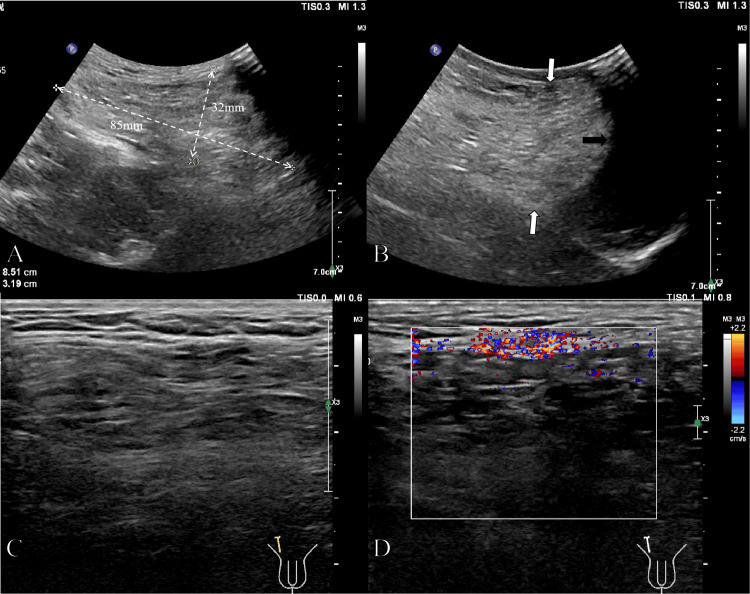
Color Doppler ultrasonography findings. A low-frequency probe was used to evaluate the overall morphology of the hernia sac (A); the inferior margin of the hernia sac (white arrow, B) extended into the scrotum (black arrow, B). High-frequency ultrasonography demonstrated linear hyperechoic structures within the hernia sac consistent with omental tissue (C), and Color Doppler imaging revealed a few intralesional blood flow signals (D).
